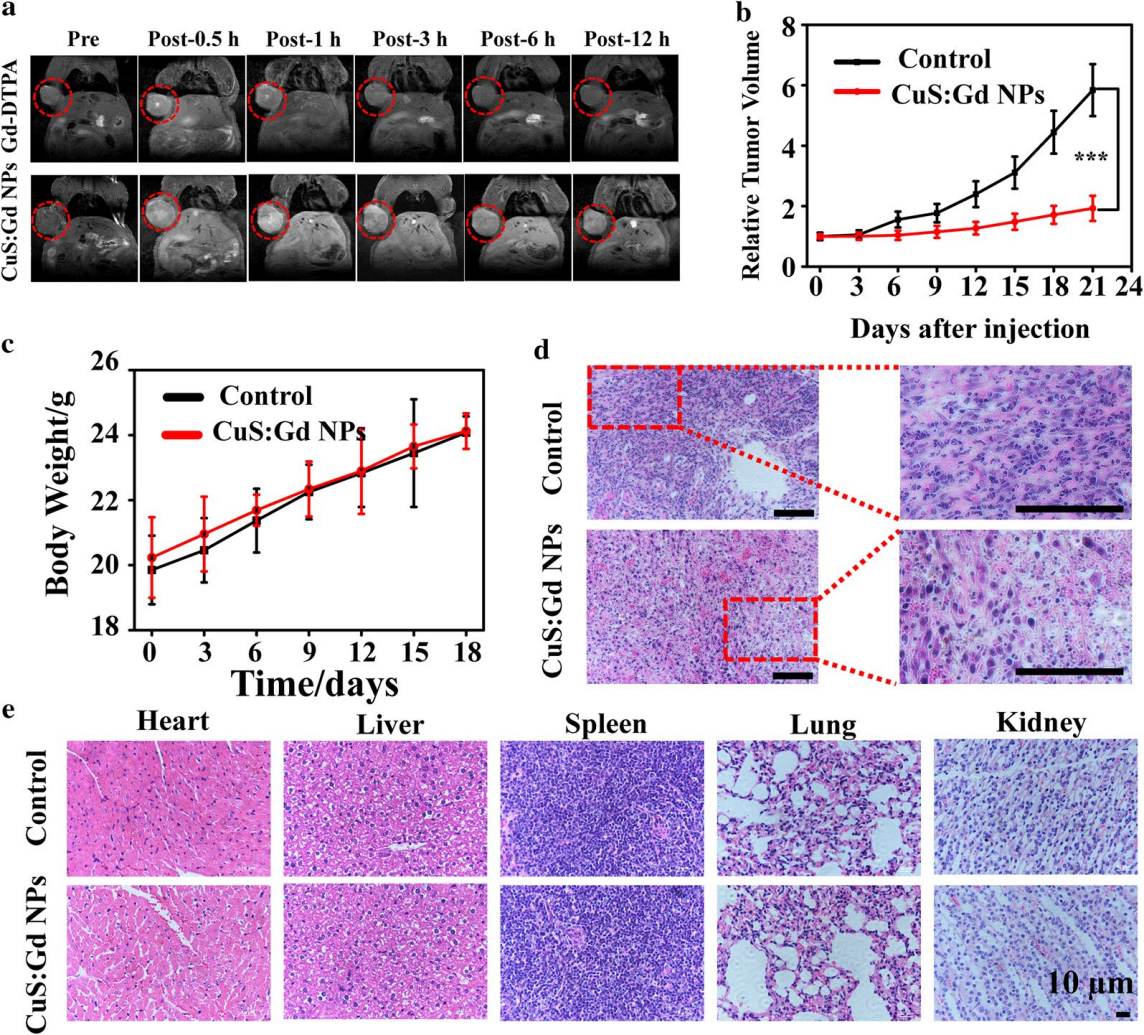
In vivo MRI performance and CDT ability of CuS:Gd NPs. a
